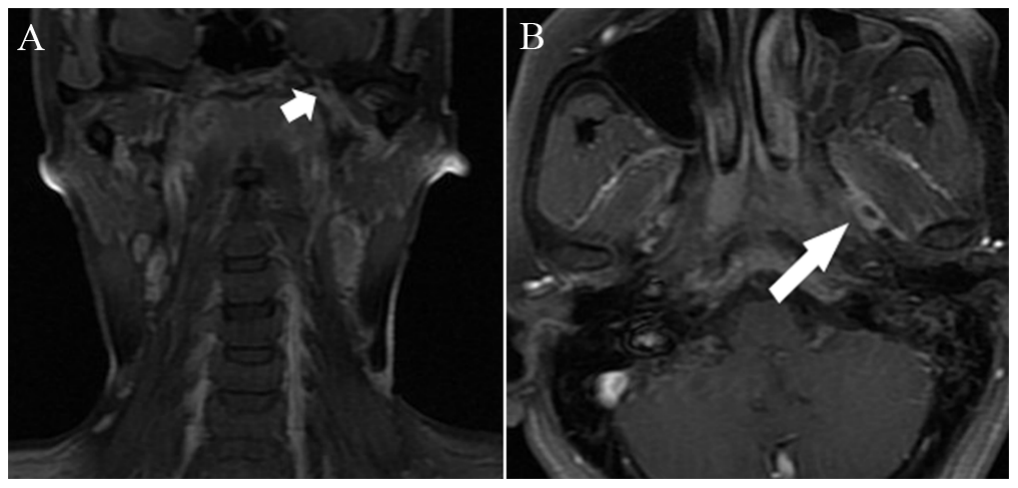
39-year-old woman with nasopharyngeal carcinoma with cranial nerve invasion: (A) enhanced coronal T1-weighted image with fat suppression shows the enlarged left foramen ovale with abnormal enhancement (short arrow); (B) the enhanced axial T1-weighted image with fat suppression shows the enlargement and abnormal enhancement of cranial nerve V3 (long arrow).
